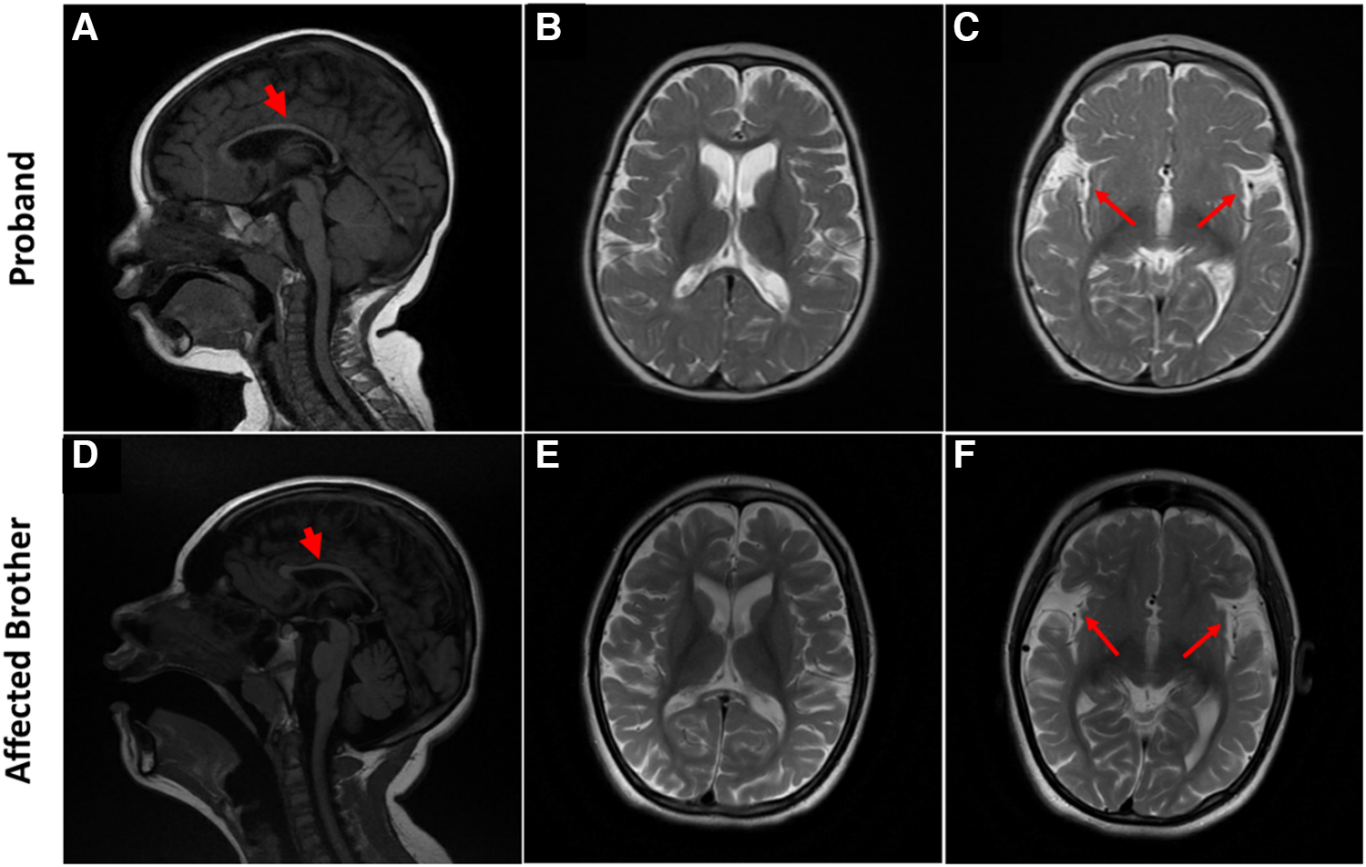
Brain MRI characteristic of SPATCCM in the proband (top panel) and his affected brother (lower panel). (A) Sagittal T1 weighted image demonstrates significant thinning of the corpus callosum. (B) Axial T2-Weighted Image demonstrating atrophy of the frontal and temporal lobes with a prominence of the subarachnoid spaces. (C) Widening of the Sylvian fissures. (D) Sagittal T1-weighted image demonstrates significant thinning of the corpus callosum and mild atrophy of the cerebellum. (E) Axial T2-Weighted Image demonstrating generalized atrophy of the frontal, temporal, and parietal lobes with a prominence of the subarachnoid spaces; enlargement of the ventricular system is not compatible with the chronological age of the patient. (F) Widening of the Sylvian fissures.
